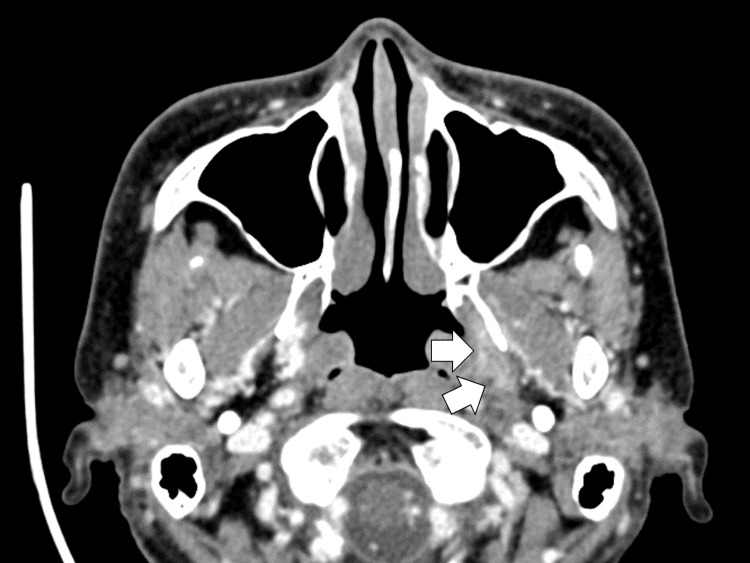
The contrast-enhanced head CT scan showed the shrinking of the left parapharyngeal abscess.
